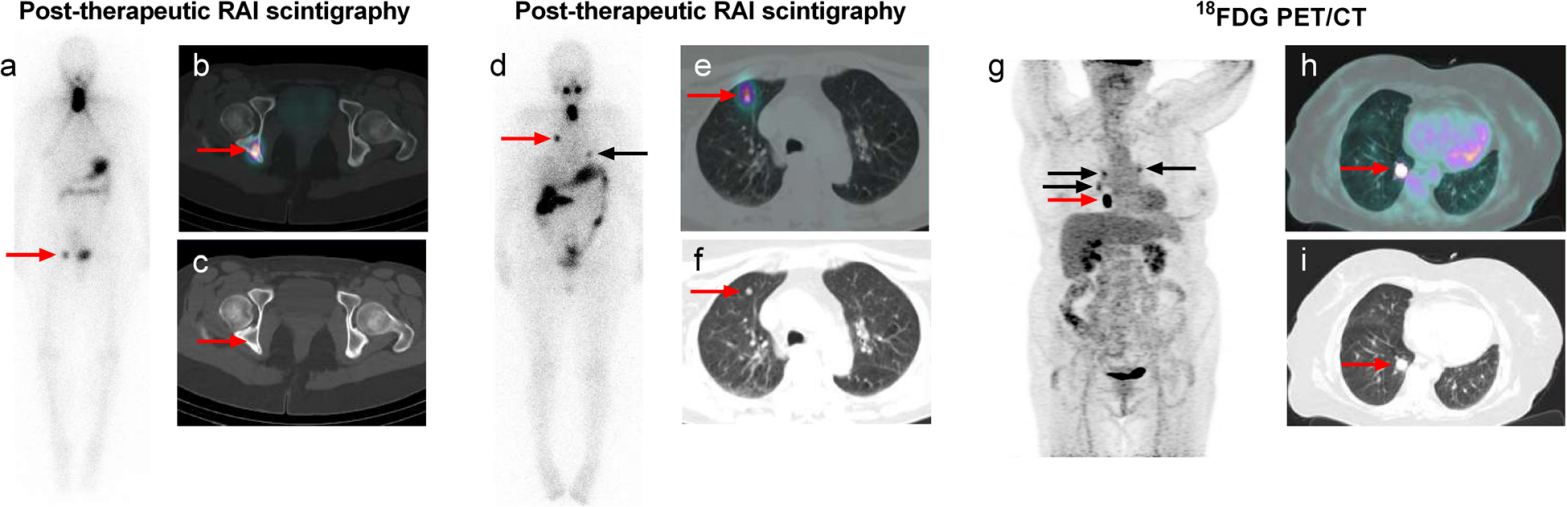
Examples of very small, small and large tumor burden in patients with persistent disease (PD). On the left side, a 43-year-old female patient with a 40-mm PTC at low-risk after initial surgery (T2NxMx) and very small-volume PD (a-c): post-therapeutic ^131^I WBS showed a solitary bony focus on the right hip (a, arrow). Fused transaxial image of ^131^I SPECT/CT (b, arrow) confirmed the bony uptake and hybrid CT (c, arrow) did not display any bone abnormality. On the middle part, a 74-year-old female patient with a 40-mm PTC at low-risk after initial surgery (T2N0Mx) and small-volume PD (d-f): post-therapeutic ^131^I WBS showed pulmonary metastases (d, red and black arrows). Fused transaxial image (e, red arrow) and hybrid CT scan (f, red arrow) depicted RAI-avid lung micronodules (e-f: 6 mm). On the right side, an 88-year-old female patient with a 40-mm PTC (tall cell variant) at high-risk after initial surgery (T2N1bM1) and large-volume PD (g-i): no abnormal RAI uptake on post-therapeutic ^131^I WBS with SPECT/CT whereas ^18^FDG PET/CT showed pulmonary and mediastinal metastases (g, Maximum intensity image, arrows). Fused transaxial image (h, arrow) and hybrid CT scan (i, arrow) showed high ^18^FDG uptake (SUVmax = 30) by an 18-mm lung nodule.
